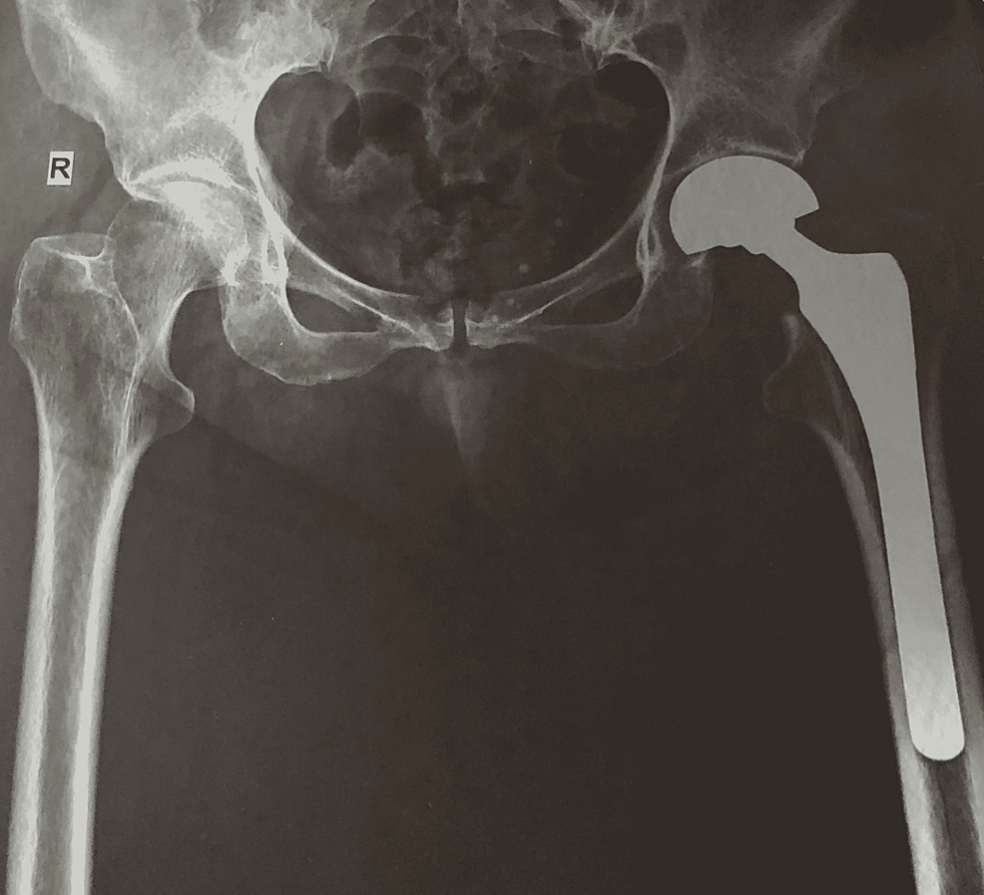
Postoperatively AP x-ray of the pelvis shows left hip hemiarthroplasty
